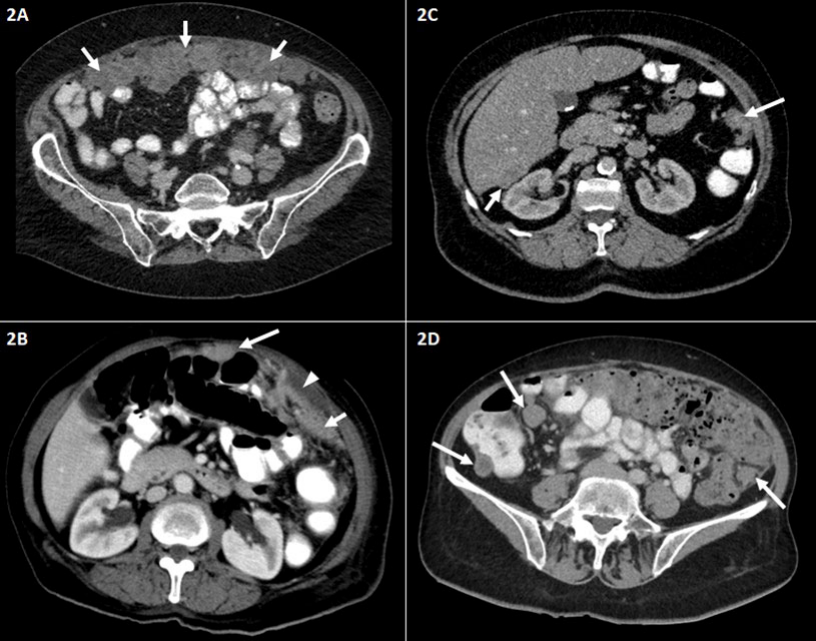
Representative abdominal CT findings in patients with advanced ovarian carcinoma FIGO stage III–IV. Axial contrast-enhanced CT scans of the middle and lower abdomen (A–D). (A) Irregular soft tissue mass representing large omental plaques called omental cake (short arrows) common site of intraperitoneal seeding of ovarian carcinoma. (B) Peritoneal thickening (short arrow) and small amount of ascites (arrowhead). Peritoneal nodule adjacent to the transverse colon (long arrow). (C) Peritoneal nodules in the left paracolic gutter (long arrow) and the hepatorenal recess (Morison’s pouch) (short arrow). (D) Peritoneal implants/nodules in the right and left paracolic gutter. FIGO, International Federation of Gynecology and Obstetrics.
